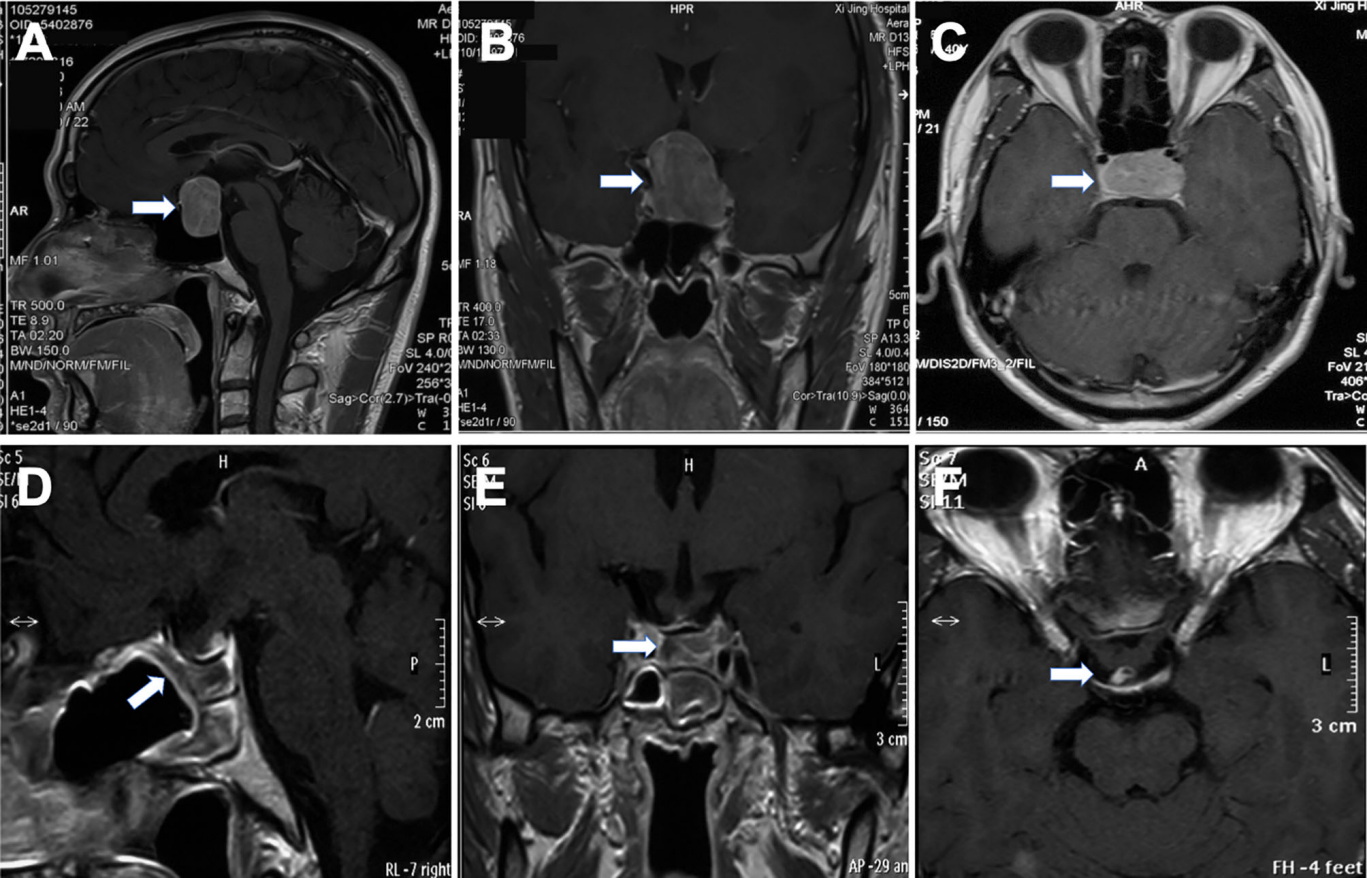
Preoperative and postoperative magnetic resonance images of pituitary adenoma. The lesion was seen in the sellar region (A, B), and the normal pituitary gland was squeezed to the right side (arrow) (C). The tumor was excised completely by EEA, mucosal flap was in good condition (D). Optic chiasm and pituitary stalk were in normal position (E, F).
